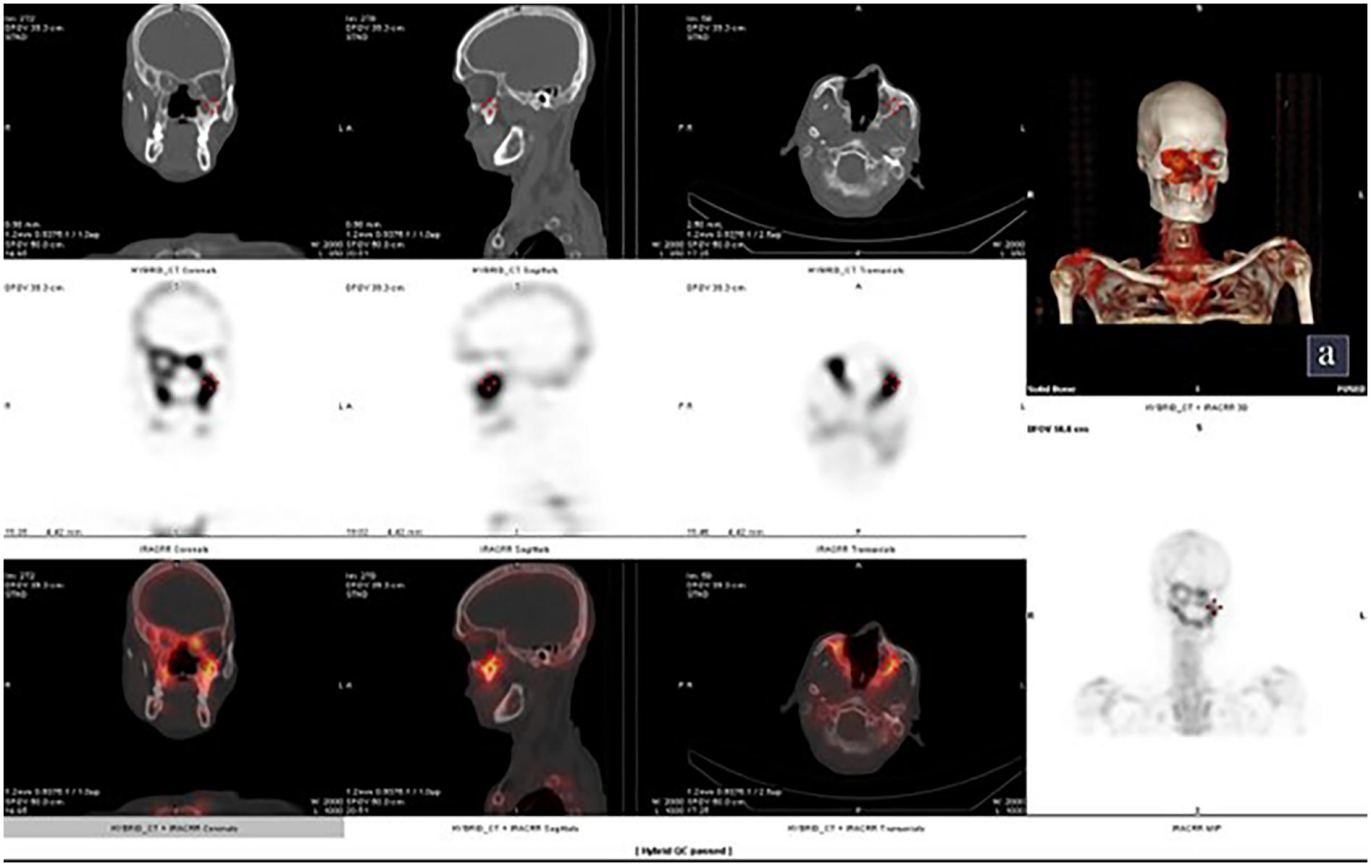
Case 3: additional SPECT/CT images showing increased tracer uptake in the lesser wing of sphenoid right side, floor and orbital plate of right maxilla, right pterygoid plate, right zygomatic bone, right mastoid bone with clouding of right mastoid air cells.
